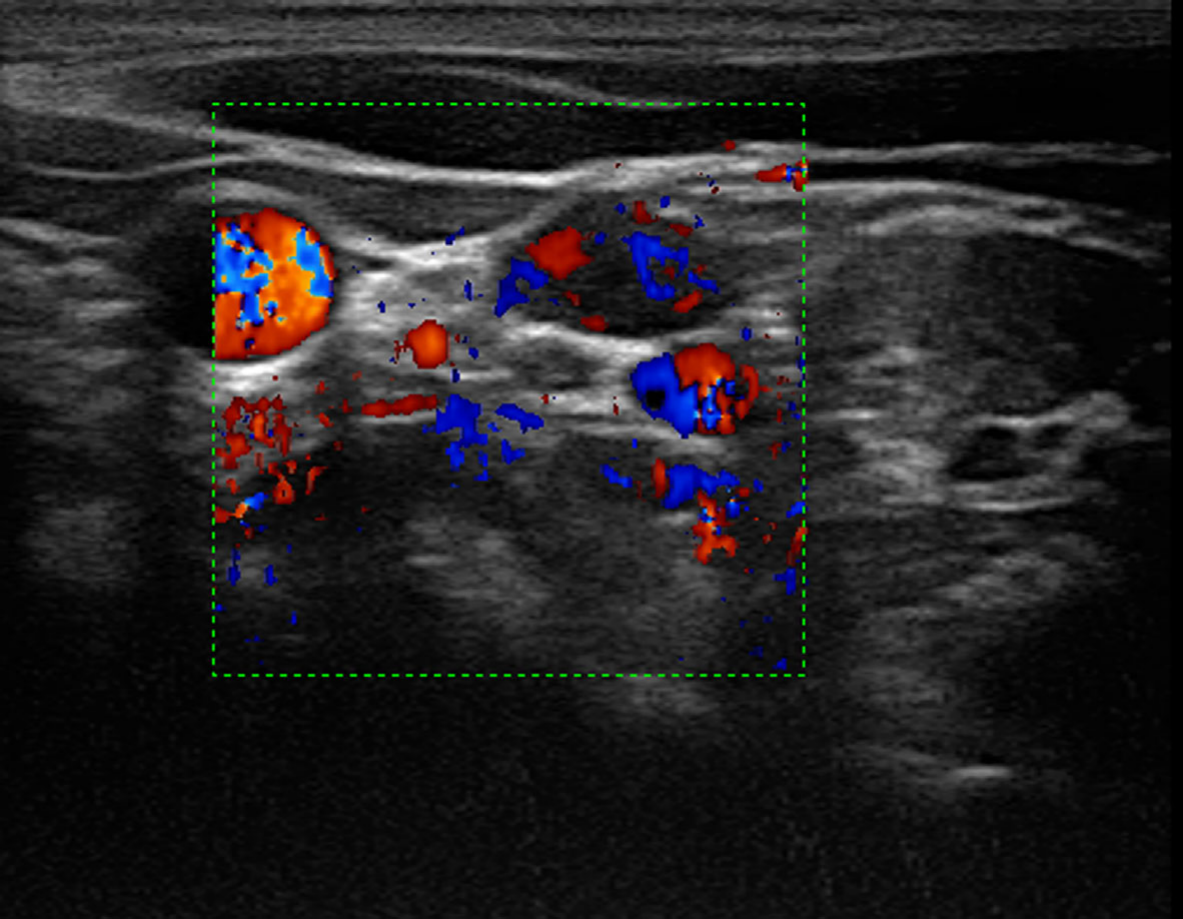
Color Doppler imaging showed rich blood supply in the lymph node. On the right side of the lymph node showed the compressed jugular vein and the carotid artery.
